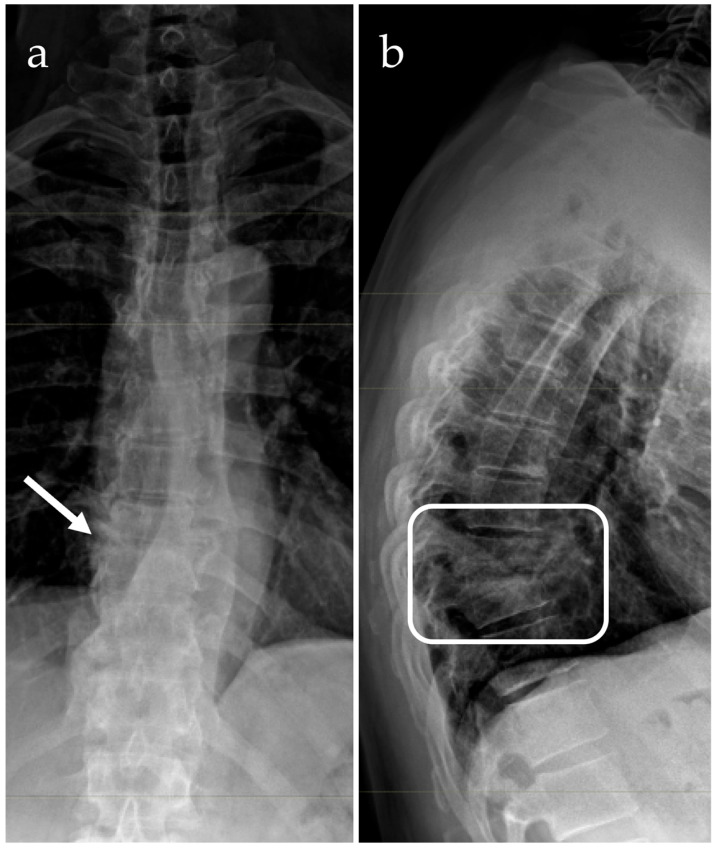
A plain X-ray of the thoracic spine in a male patient with bacterial spondylodiscitis, antero-posterior (a) and lateral (b) view. Mild T9 and T10 vertebral collapse and reduced disc height (arrow in (a) and white case in (b)).
